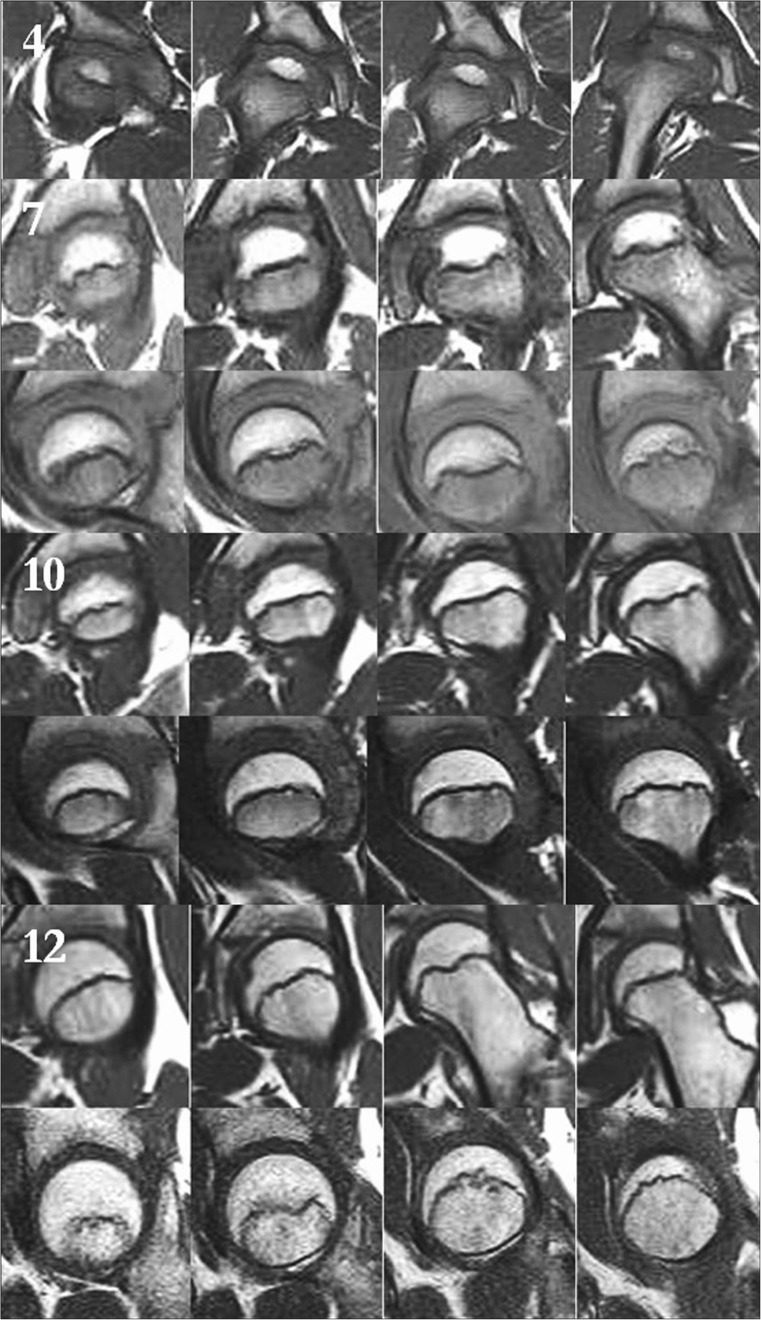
MRI scans of a growth plate of the proximal femoral epiphysis in a 4-, 7-, 10- and 12-year-old child
